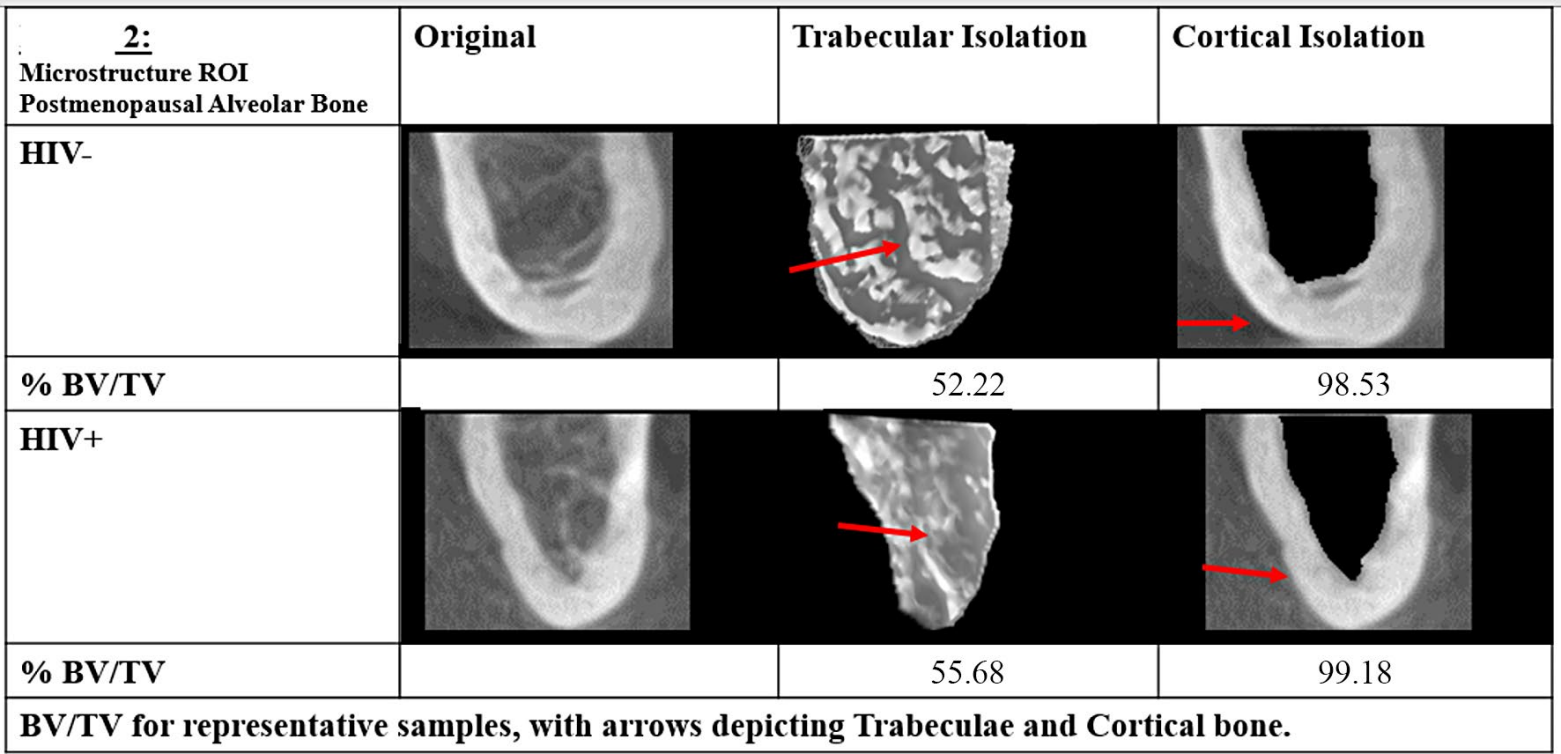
Representative Cone Beam 3-D images of the original mandibular alveolar bone and the trabecular and cortical compartments from people with HIV (PWH) and HIV-negative controls
